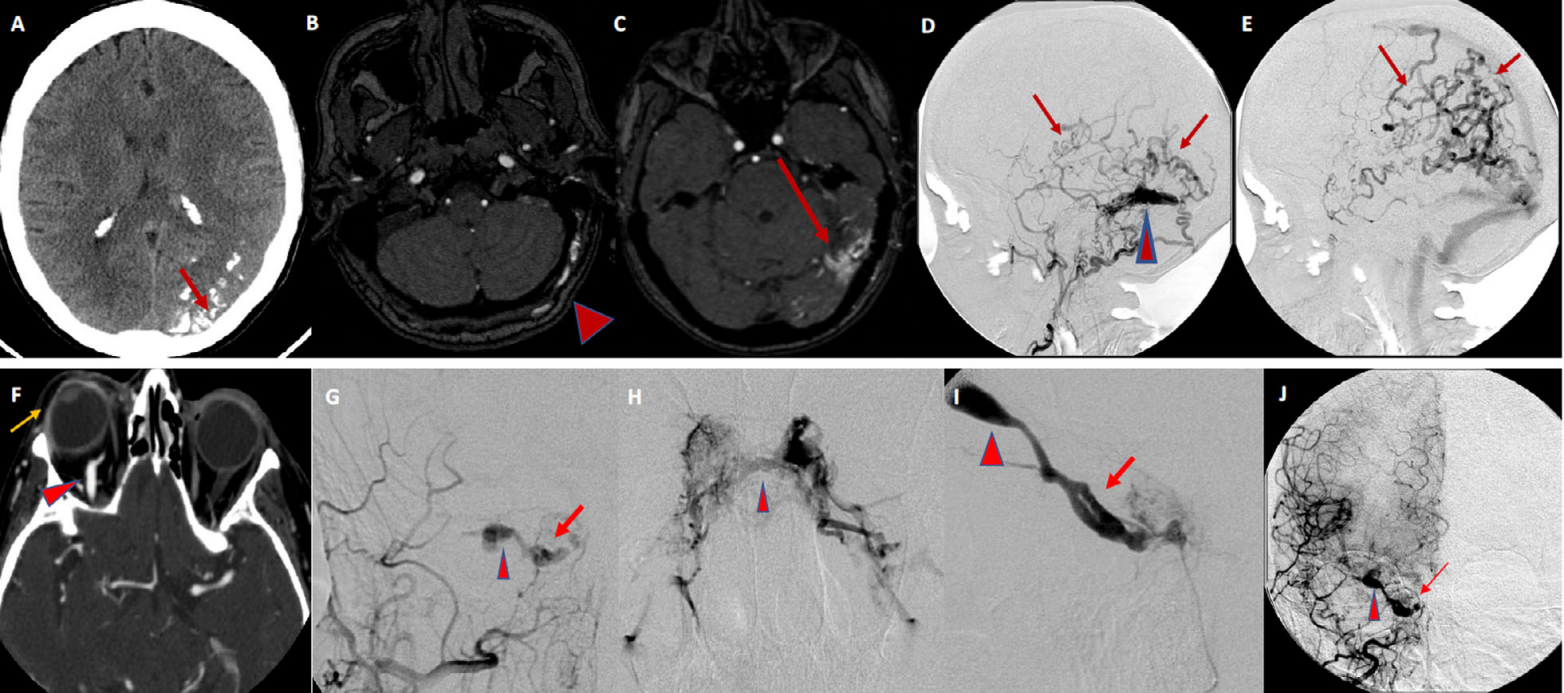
A middle‐aged man with seizure, headache, and right visual field disturbance. Axial noncontrast head CT demonstrating calcification in the walls of the left occipital veins (arrow) indicating long‐standing venous hypertension (A). Axial images from noncontrast MRA head, demonstrating an asymmetrically enlarged left occipital artery (arrowhead). Arterialized veins (arrows) are seen in the left occipital and temporal lobes (B, C). Lateral views of left occipital artery angiogram demonstrating a venous recipient pouch in the left transverse sinus wall (arrowhead) with retrograde cortical venous drainage (arrows) (D, E). Imaging consistent with high‐grade dural arteriovenous shunt. A 77‐year‐old woman presenting with swollen preseptal soft tissues, proptosis, conjunctival injection, and eye pain. Axial reconstructions of a CTA Head demonstrating right eye proptosis (yellow arrow) and an asymmetrically enlarged and enhancing superior ophthalmic vein (arrowhead). Lateral views of the right internal carotid angiogram show an indirect CCF, with opacification of the superior ophthalmic vein (arrowhead) and right Cavernous sinus (arrow), with the absence of the ipsilateral inferior petrosal sinus (IPS), including on the normal venous phase of the brain (star denotes expected location).
